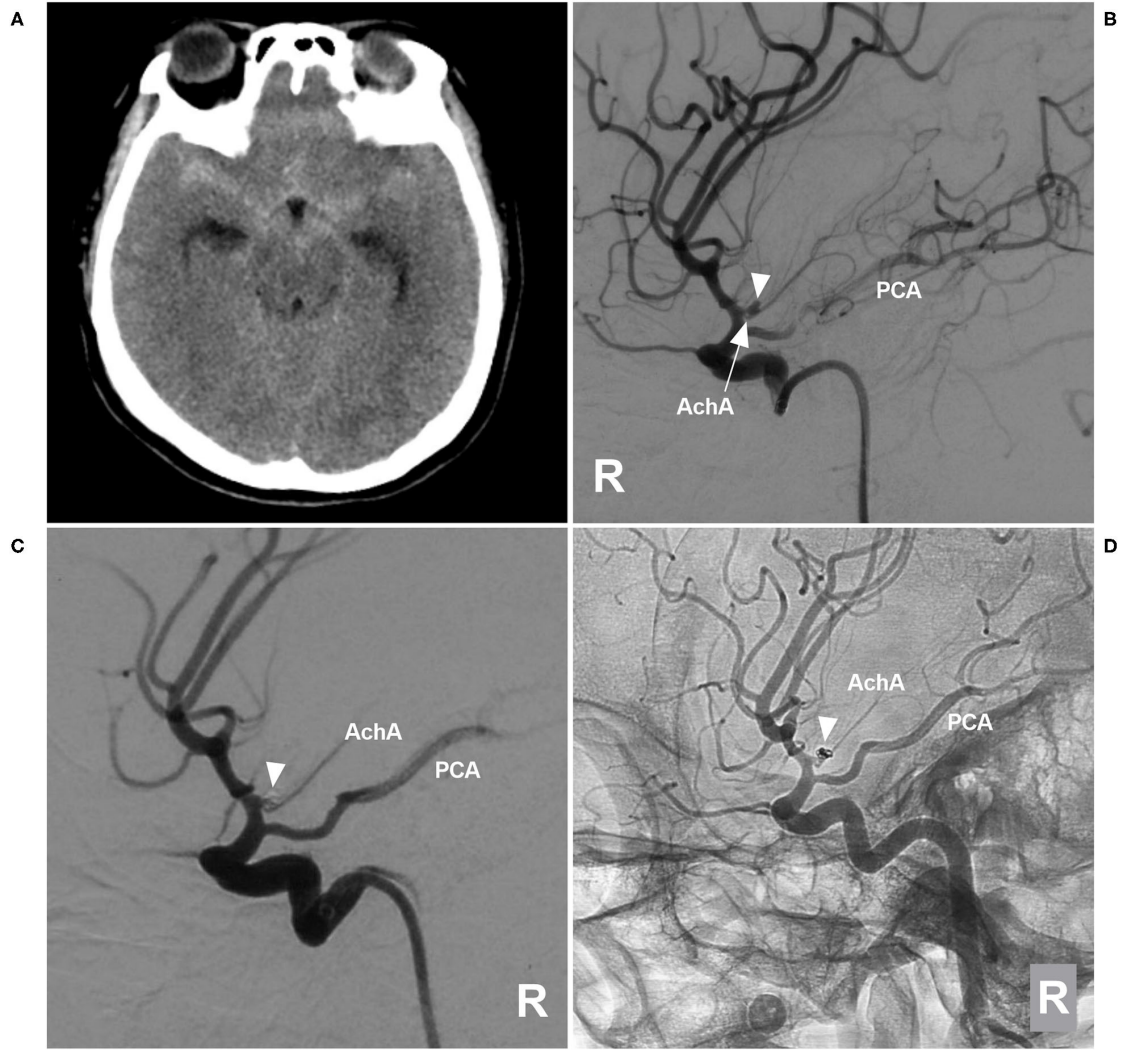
EVT of a type II AchA aneurysm. (A) CT showing subarachnoid hemorrhage. (B) DSA of the right ICA showing a type II AchA aneurysm (triangle); the AchA (arrow) arose from the aneurysm neck, and the PCA was the fetal type. (C) DSA of the right ICA showing that the aneurysm (triangle) was coiled, with MRCC grade I, the AchA was unblocked. (D) Follow-up unsubtracted DSA of the right ICA showing that the aneurysm (triangle) had no recurrence. AchA, anterior choroidal artery; CT, computed tomography; DSA, digital subtraction angiography; EVT, endovascular treatment; ICA, internal carotid artery; MRCC, Modified Raymond-Roy Classification; PCA, posterior cerebral artery; R, right; 3D, three-dimensional.
